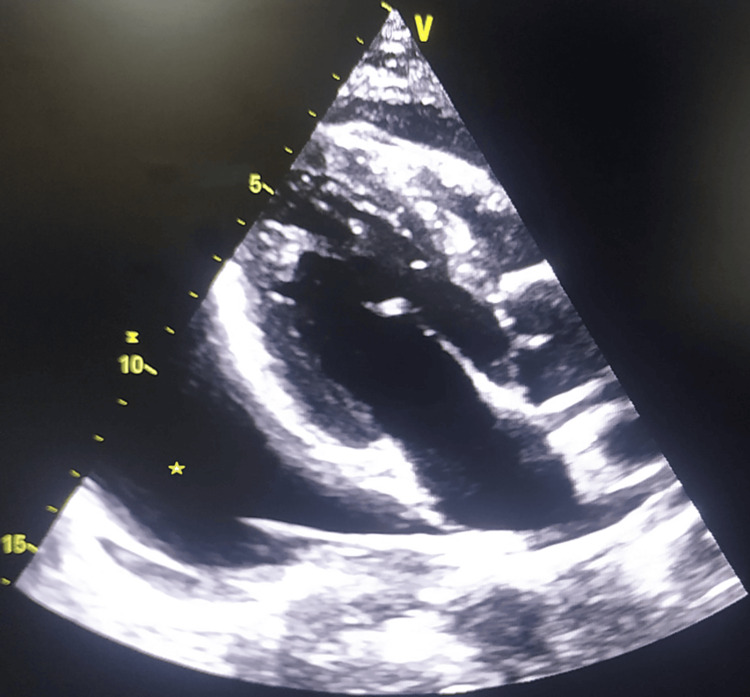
2D echocardiography image in parasternal long axis view showing massive pericardial effusion (asterisk)
